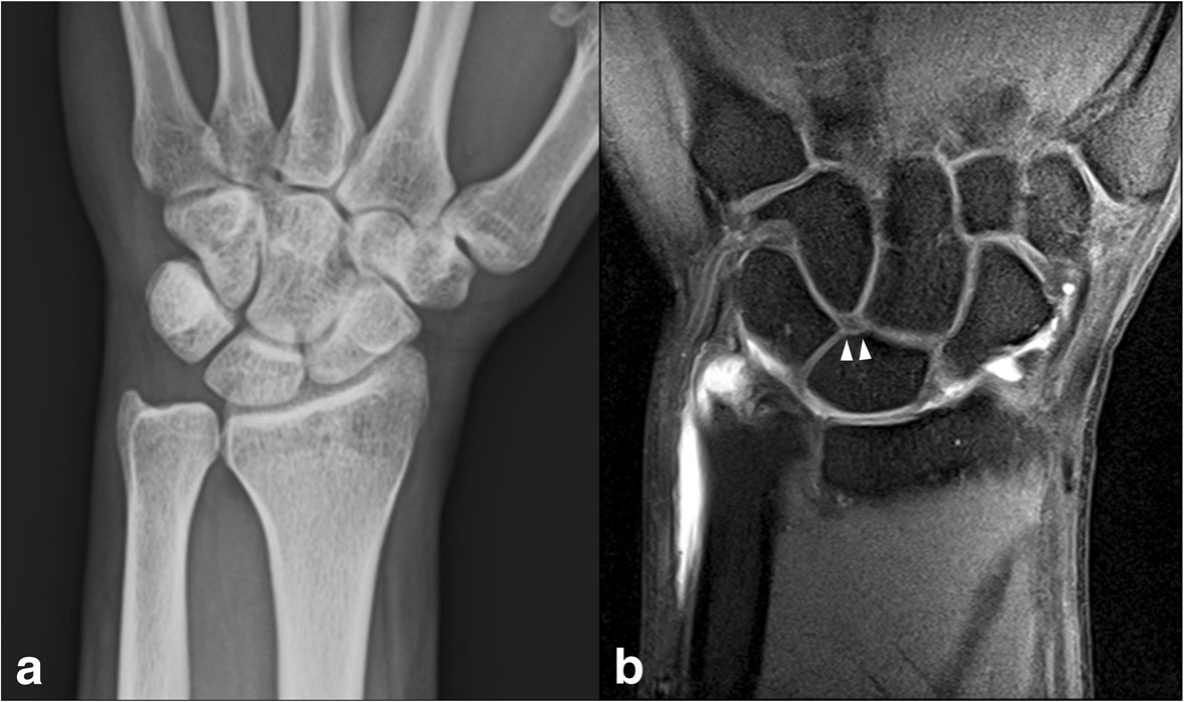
A 37-year-old man with wrist pain. (a) Wrist plain radiograph recorded as Type I lunate on plain radiographic analysis. (b) Coronal fat-saturated T1-weighted arthrographic image showing a radiographically undetected medial facet of the lunate (arrowhead)
